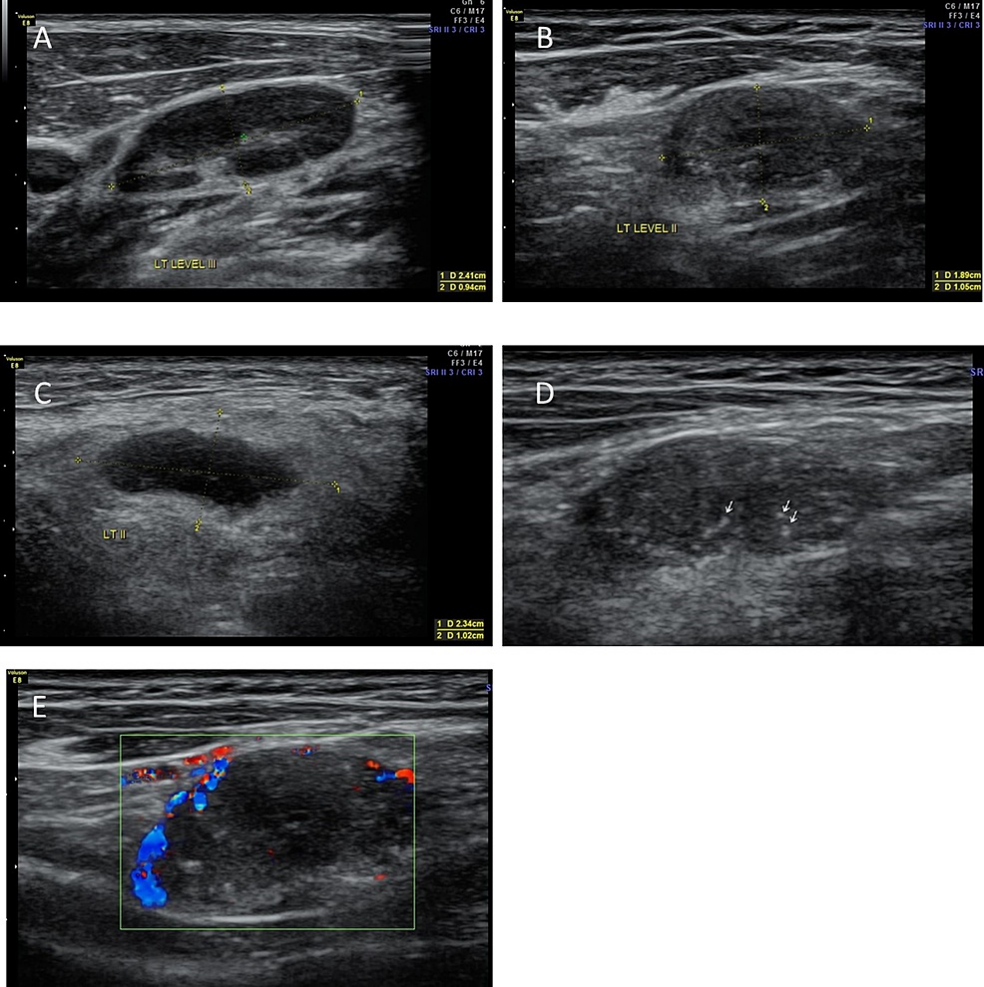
B-mode (A-D) and Doppler (E) ultrasonography images of cervical lymph nodes. (A) B-mode ultrasonography showing short-axis dimension more than 0.8 cm and normal hilum; (B) B-mode ultrasonography lymph node showing short-axis dimension more than 0.8 cm and short-axis to long-axis diameter ratio of more than 0.5; (C) B-mode ultrasonography showing enlarged hypoechoic lymph node with absent hilum; (D) B-mode ultrasonography showing calcifications (white arrows) in lymph node; (E) ultrasound doppler study showing predominantly peripheral vascularity.
